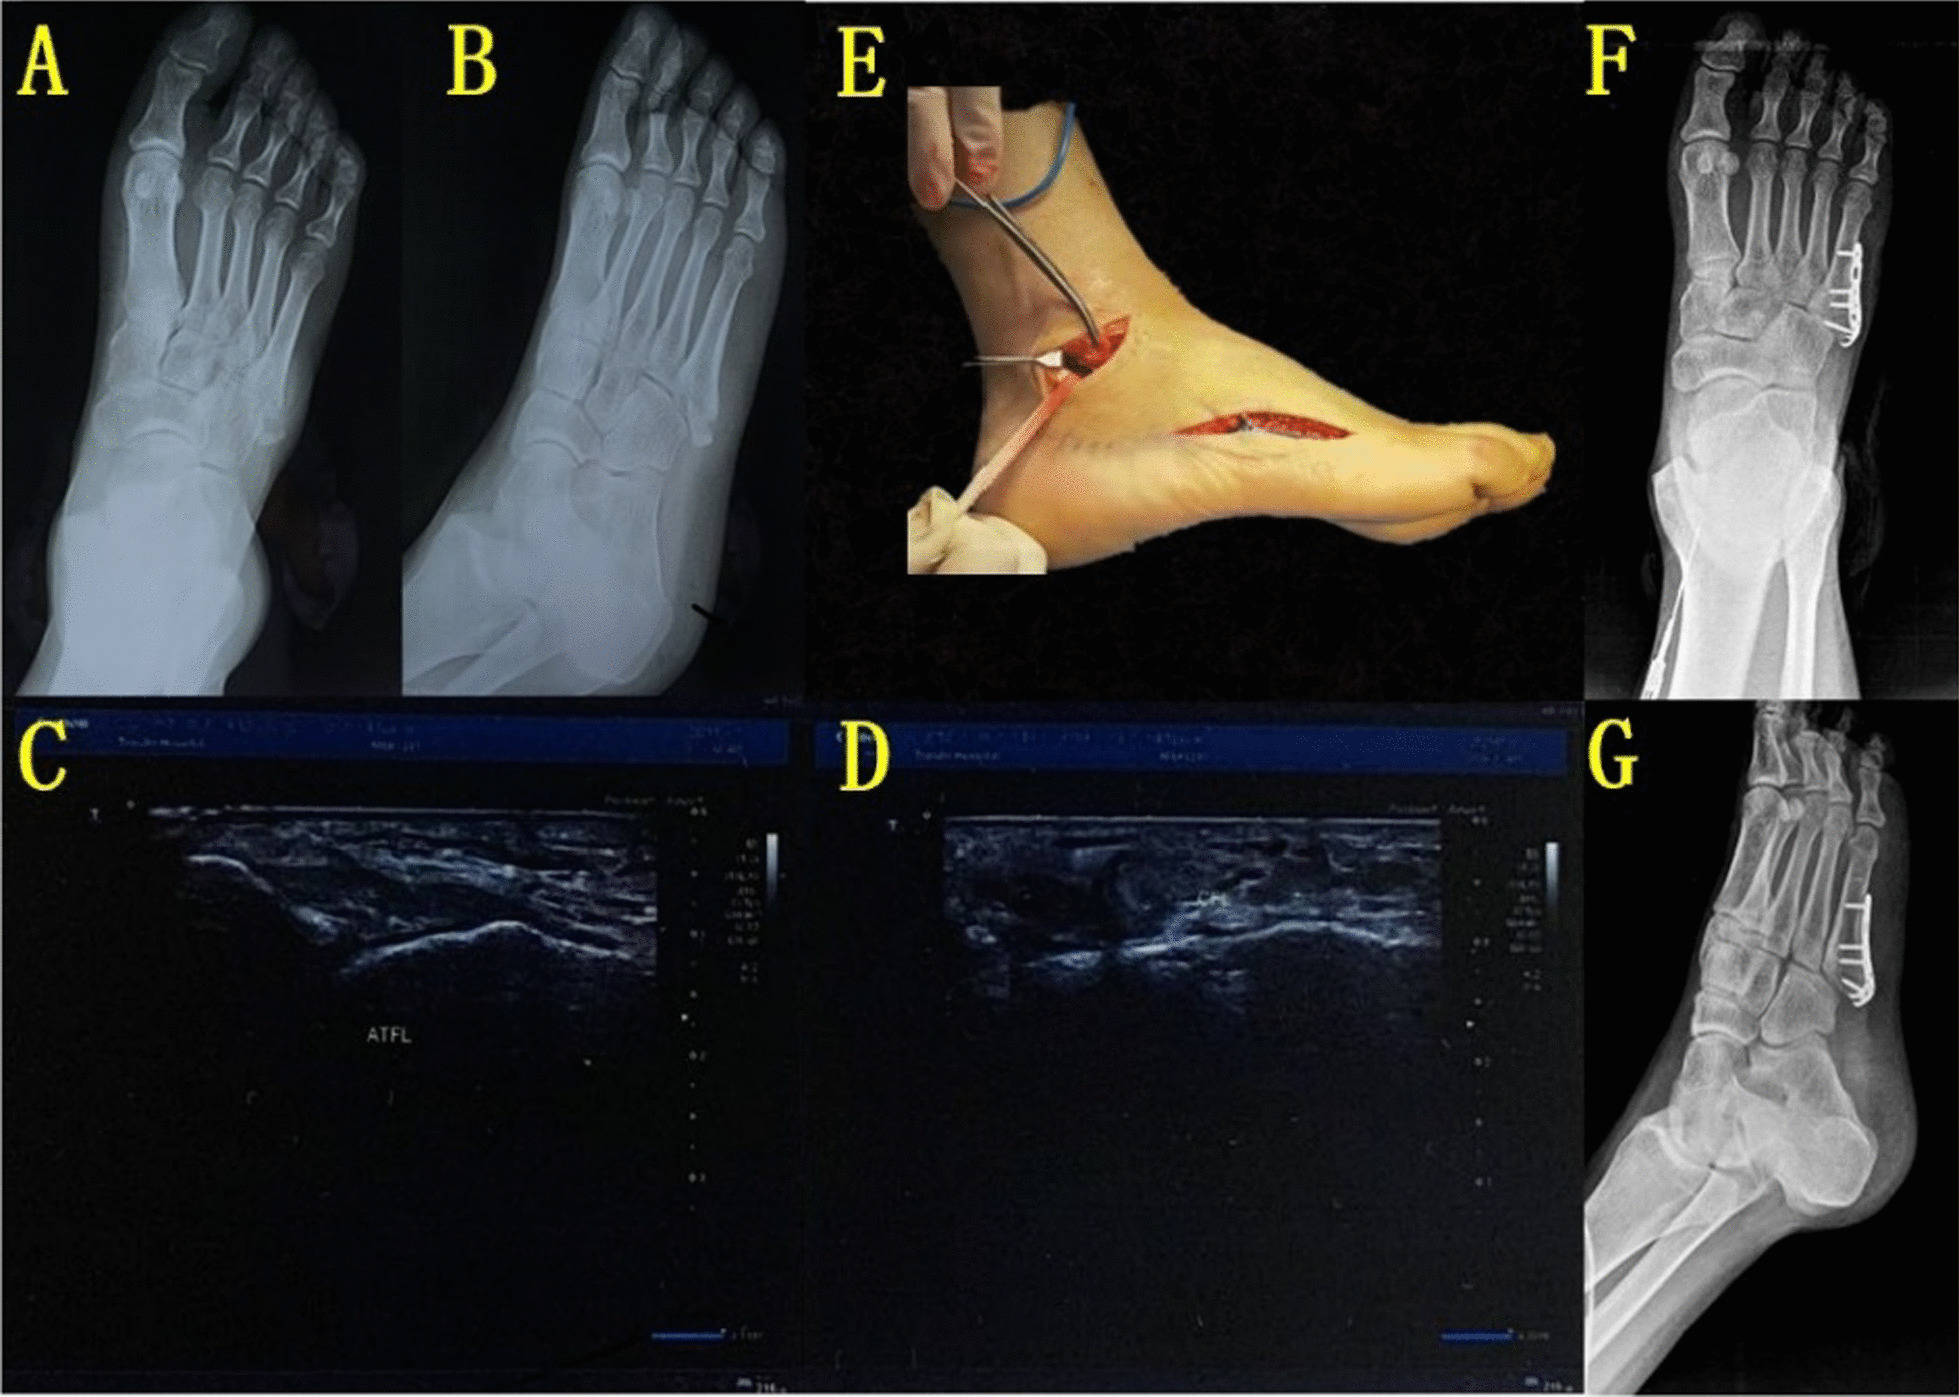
A, B Preoperative foot radiographs showed displaced-fifth metatarsal base fracture, arrow showed fifth metatarsal base fracture. C, D Preoperative ultrasonic examination showed partial injuries of anterior talofibular ligament and calcaneofibular ligament. E Intraoperative exploration showed calcaneofibular ligament completely rupture, but anterior talofibular ligament continuously exists with acceptable tension, arrow showed calcaneofibular ligament. F, G Postoperative foot radiographs showed that the fifth metatarsal base fracture was fixed with a hook plate, and the fracture was anatomically reduced
